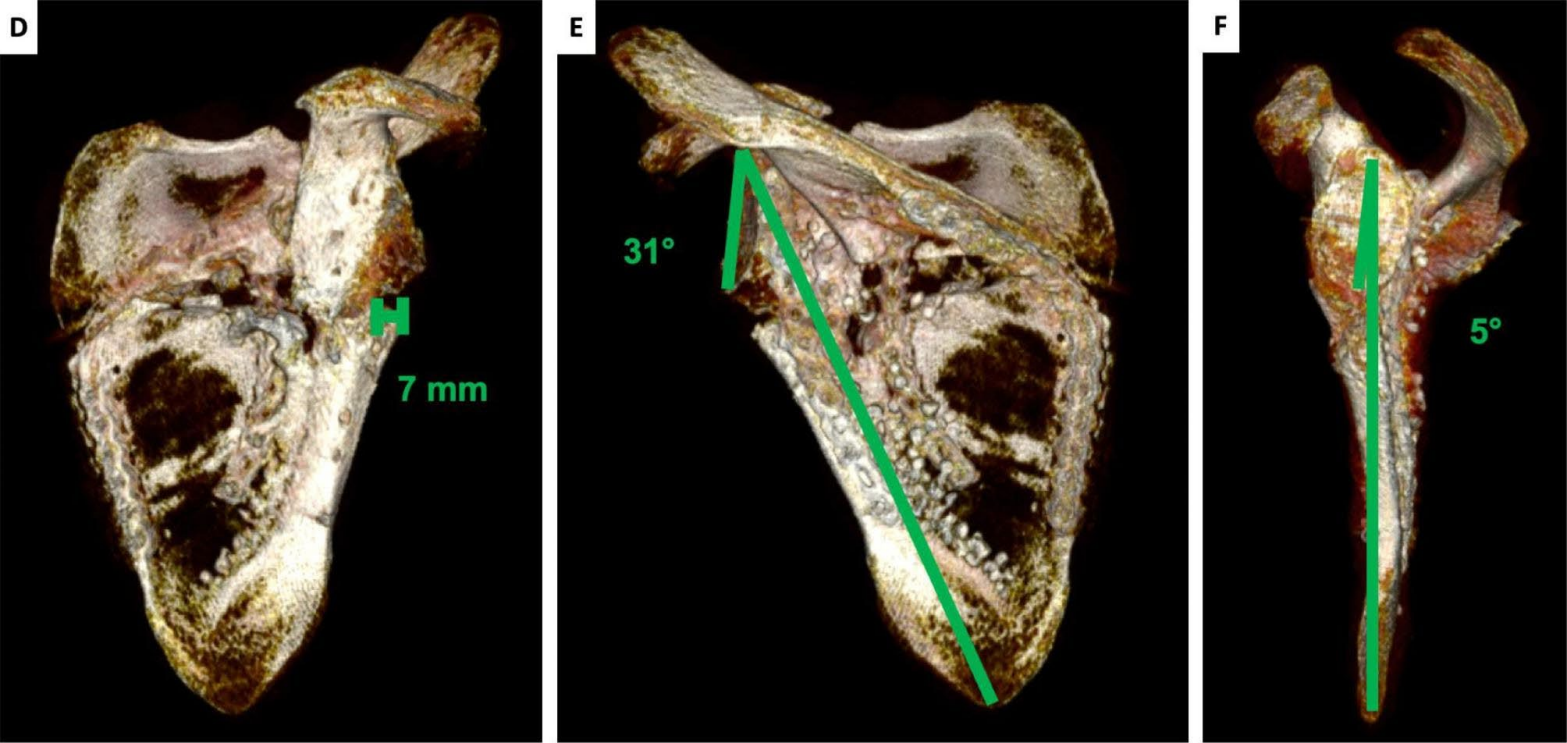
CT scan after surgery. A: lateral border offset 7 mm. B: glenopolar angle 31°. C: angulation deformity 5°
